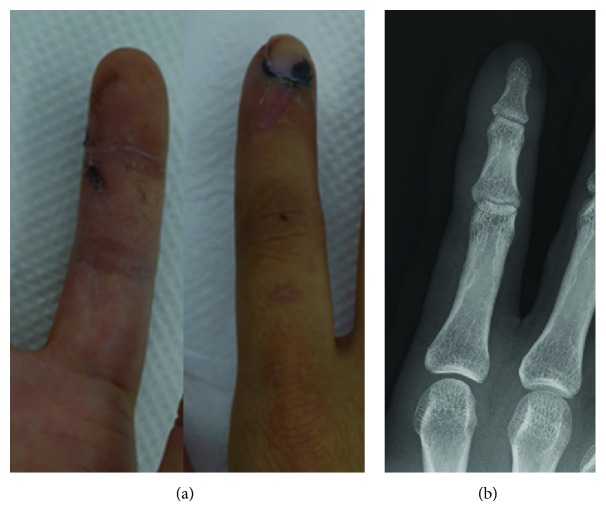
Macro and radiological findings. (a) Macrofinding at 2 weeks after injury. A scar around the ulnar side of the distal interphalangeal (DIP) joint and submaxillary hematoma in index finger was observed. (b) X-ray finding. No fracture was observed.
